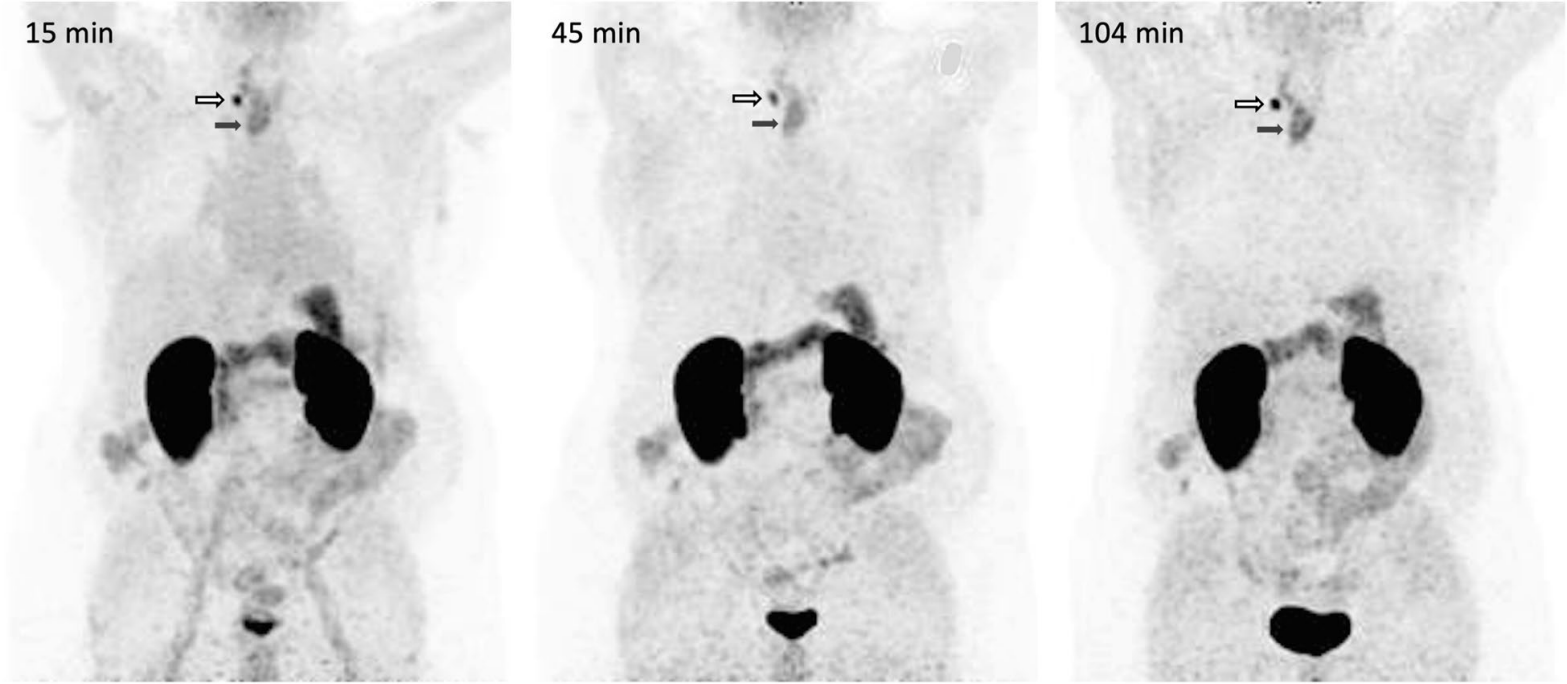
^68^Ga-D0103 PET (MIPs, scaled to SUV 15) of a patient with esophageal squamous cell carcinoma (ESCC; f, 68 y, 115 kg, 193 MBq). Times indicate scan starts after tracer injection (p.i.). Solid arrows: ESCC primary tumor. Outline arrows: lymph node metastasis
